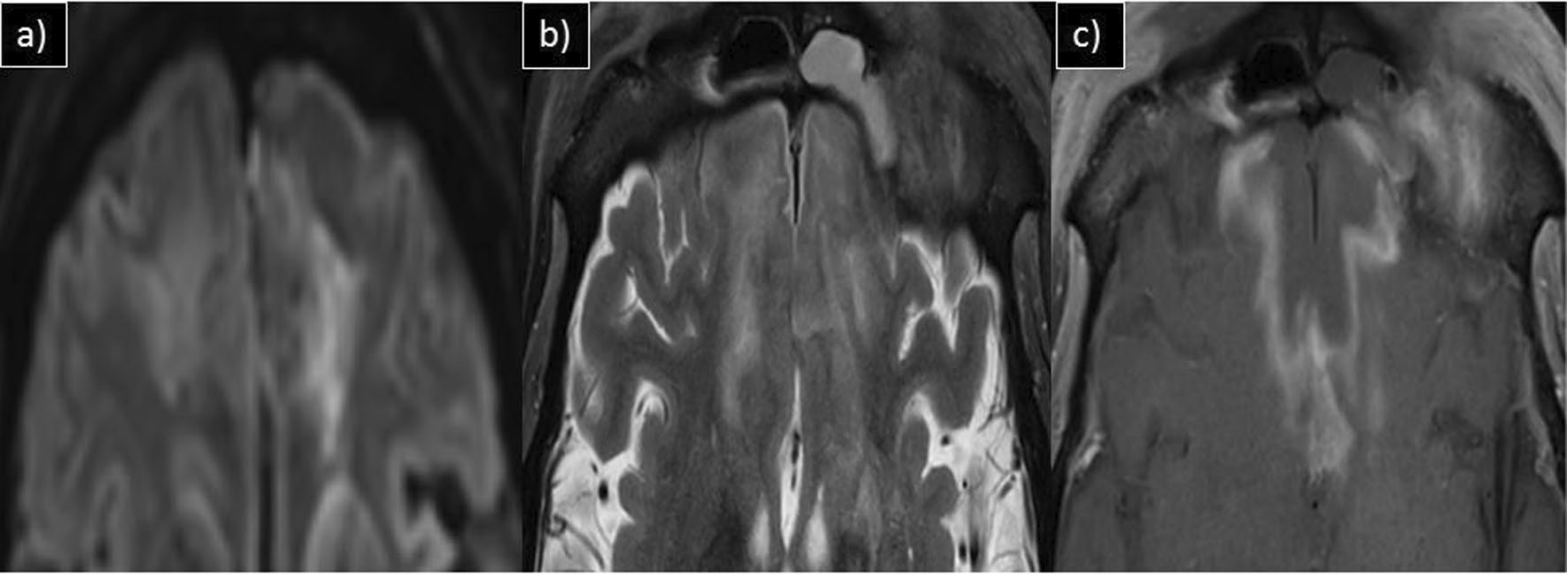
a MRI axial iso-DWI, b axial T2W-FS and c axial post-contrast T1W-FS images of a 37-year-old male patient with complaints of headache and altered sensorium showing features of late cerebritis in bilateral frontal lobe. Left frontal sinusitis is noted
